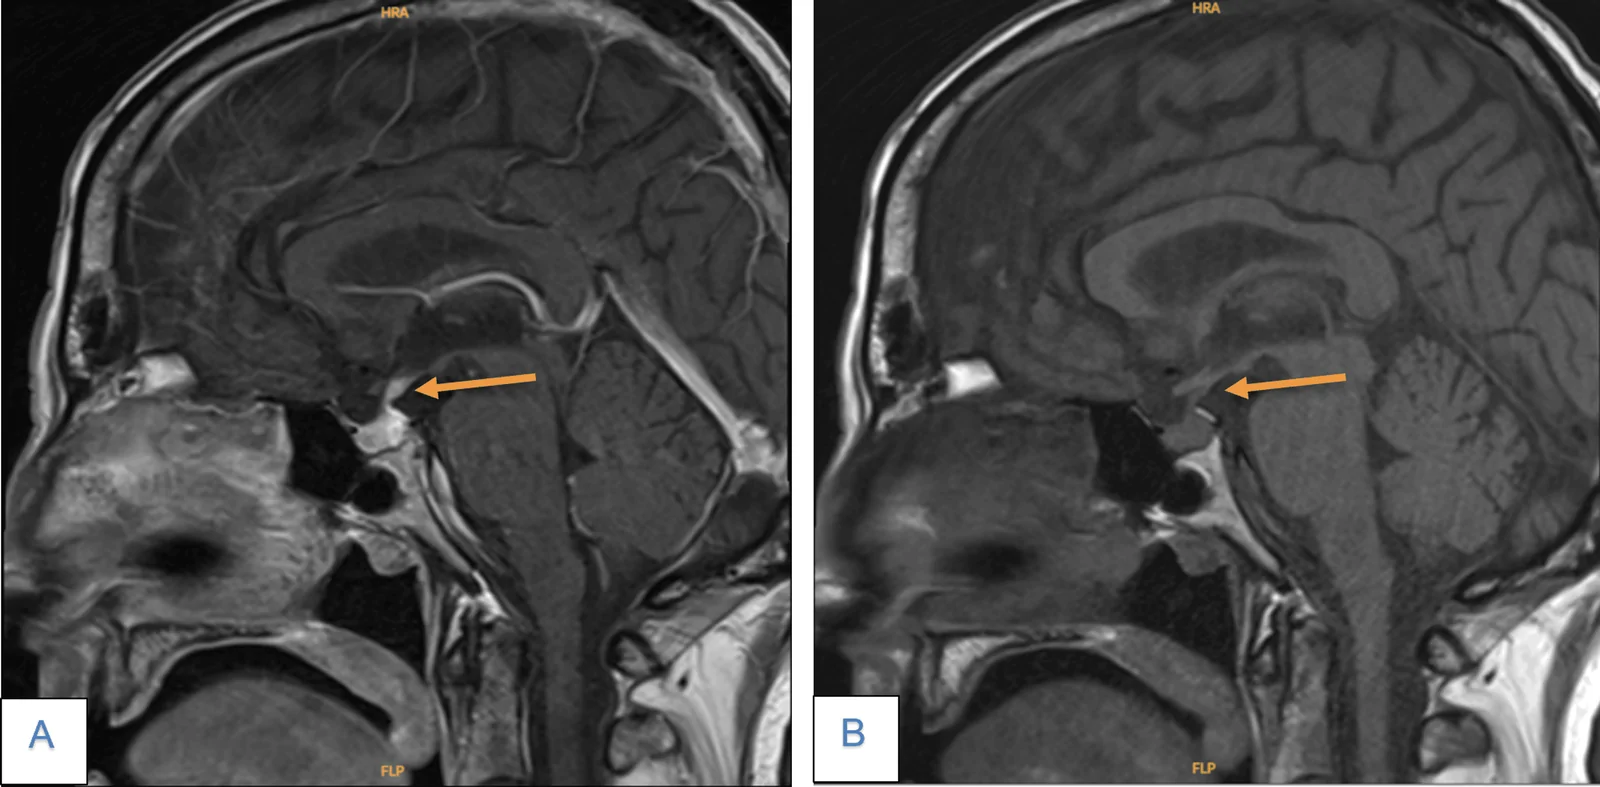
Sagittal magnetic resonance imaging (MRI) of the pituitary region (A) Post-contrast sagittal T1-weighted MRI demonstrating thickening and enhancement of the pituitary stalk. The orange arrow indicates the area of pituitary stalk thickening and enhancement.
